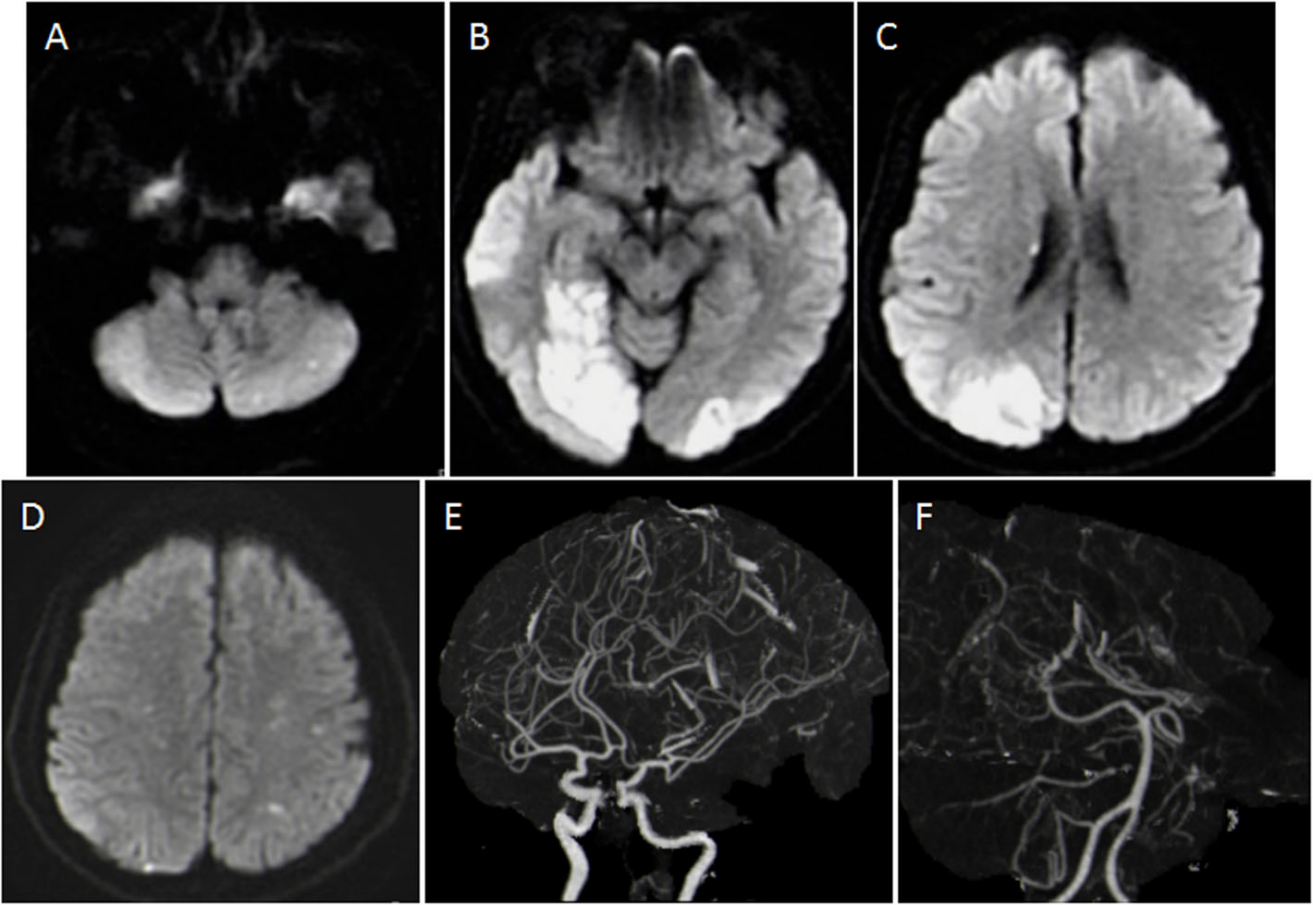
DWI showed multiregional cerebral infarction in “three territories” (a-d). Brain CTA images showed no stenosis and occlusion in cerebral arteries (e and f)
